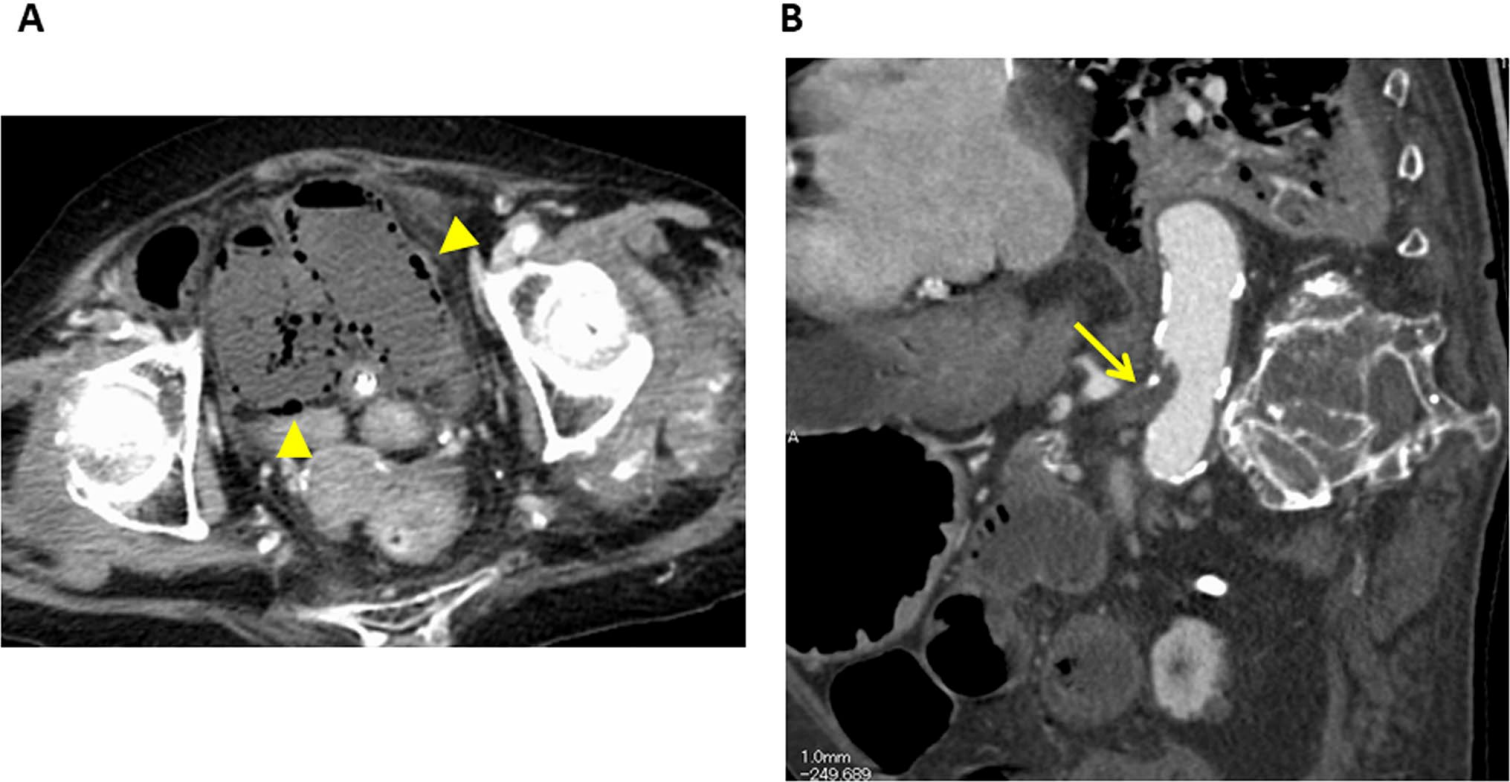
Superior mesenteric artery embolism in an 80-year-old woman with a history of a thrombus in the auricle of the left atrium. A Axial contrast-enhanced CT image (venous phase) revealing gas in the bowel wall (arrow heads) (pneumatosis intestinalis). B Oblique sagittal contrast-enhanced CT image (arterial phase) shows an occluding embolus (arrow) in the superior mesenteric artery
